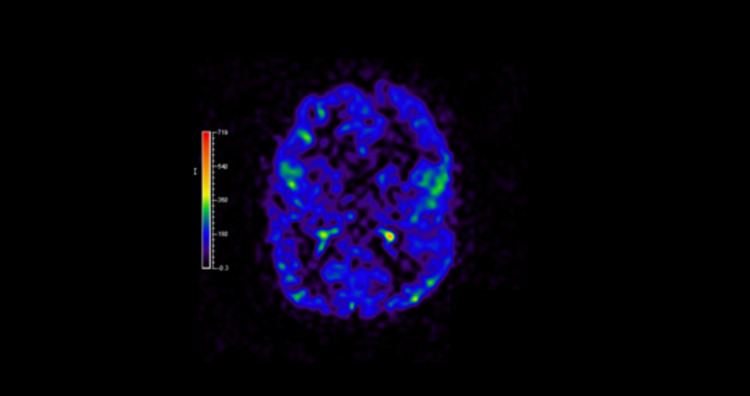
CT perfusion sequence. The scale represent areas with highest metabolism (red) and zones with lower metabolism (purple-black)
